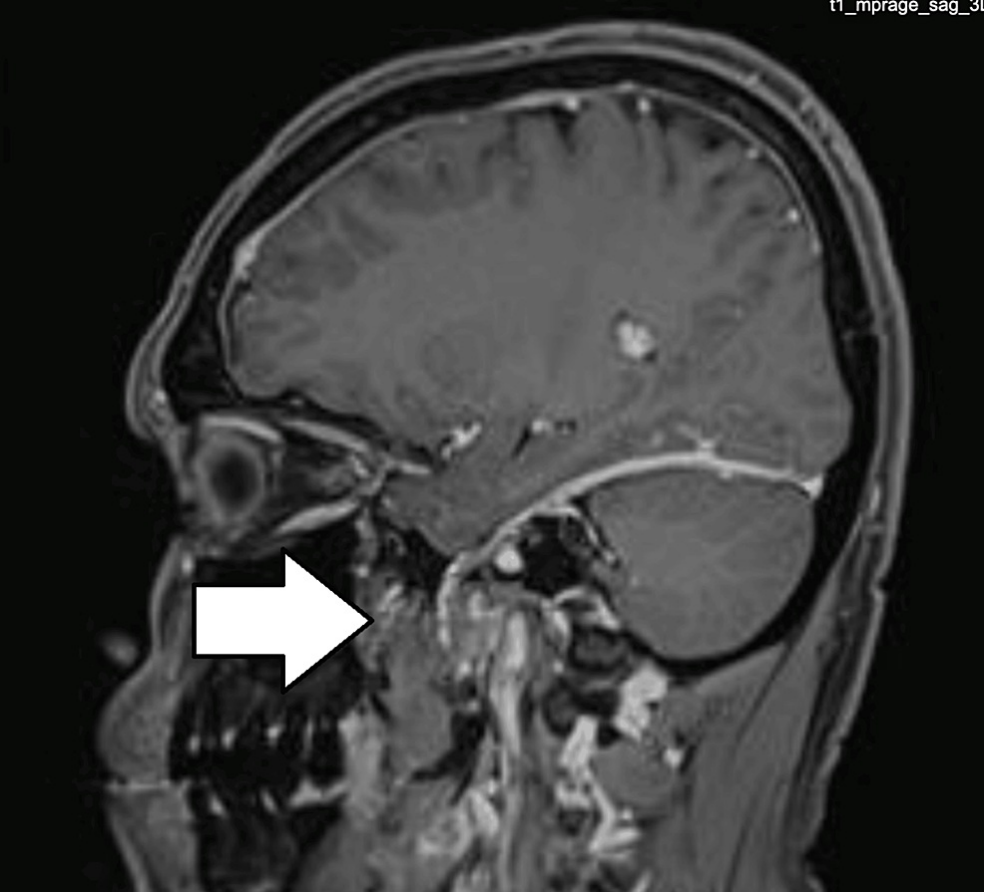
MRI of the brain: T1 contrast, sagittal view, showing contrast enhancement of the meninges
